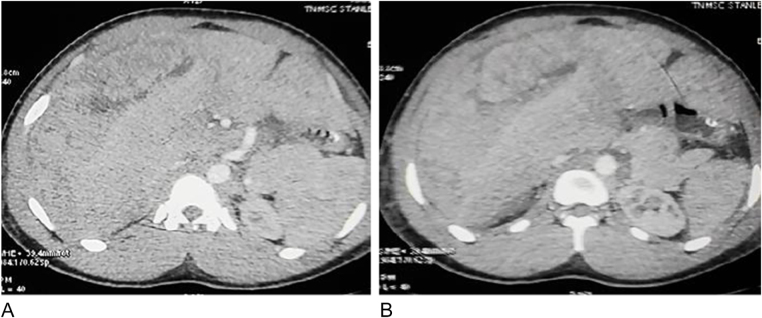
A & B: Contrast-enhanced computed tomography (CECT) of the abdomen and pelvis (early arterial phase), performed on the day of admission (07/09/2024), demonstrates a large non-enhancing subcapsular hepatic hematoma (21 × 5.2 × 18.3 cm) with associated hemoperitoneum. A heterodense lesion measuring 7.3 × 10.4 × 6 cm is noted in segment VII, with no evidence of active contrast extravasation.
